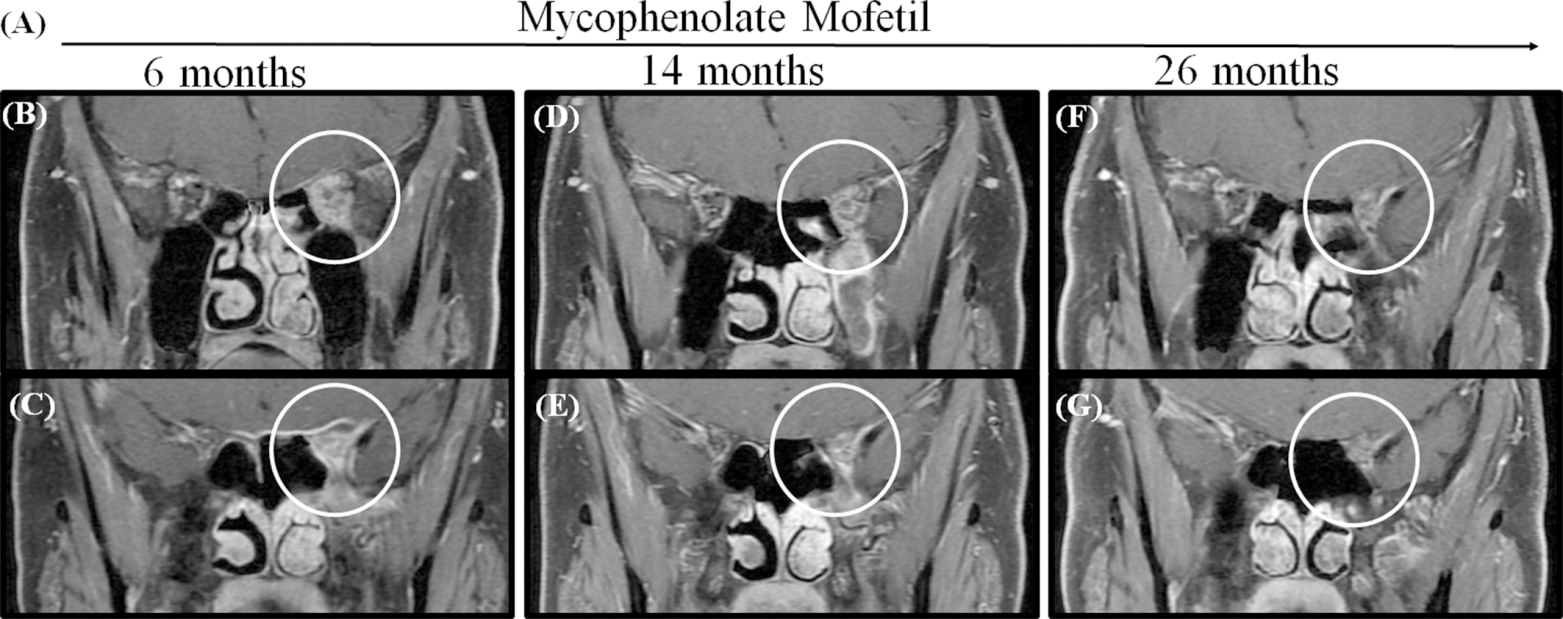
MRI shows that the lesion became gradually smaller in patient 9 when administrated with Mycophenolate Mofetil for more than 2 years. (A) The time axis of used Mycophenolate Mofetil. (B, C) MRIs obtained after using Mycophenolate Mofetil for 6 months showed a contrast-enhanced mass extending the orbital apex to the cavernous sinus (circle). (D, E) The lesion reduced in 14 months. (F, G) The lesion got smaller in 26 months but did not disappear.
